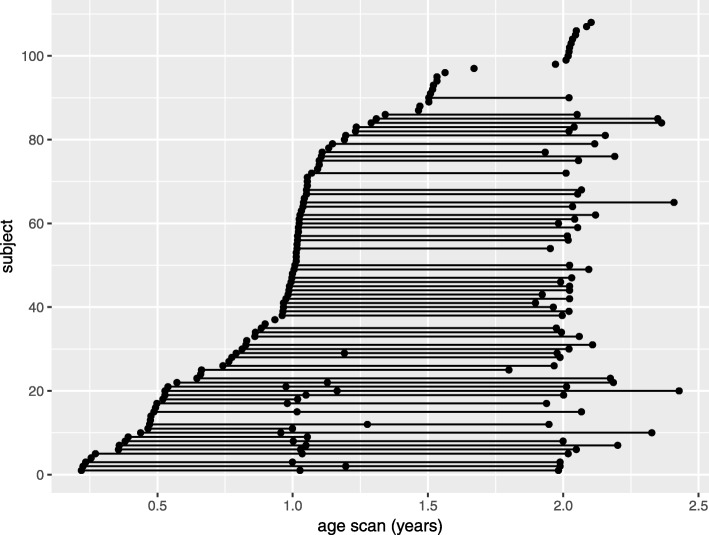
Depiction of the MRI sample. Each point represents an MRI scan. A line connecting multiple points represents repeated MRI scans for a single patient
